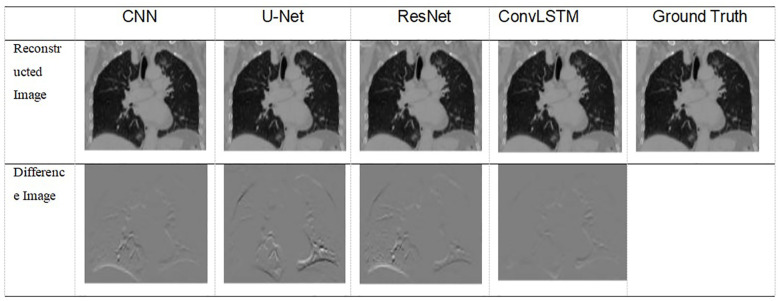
Visualization of images result of TestCase1 for each model with the training data generated from 4D-CT.
